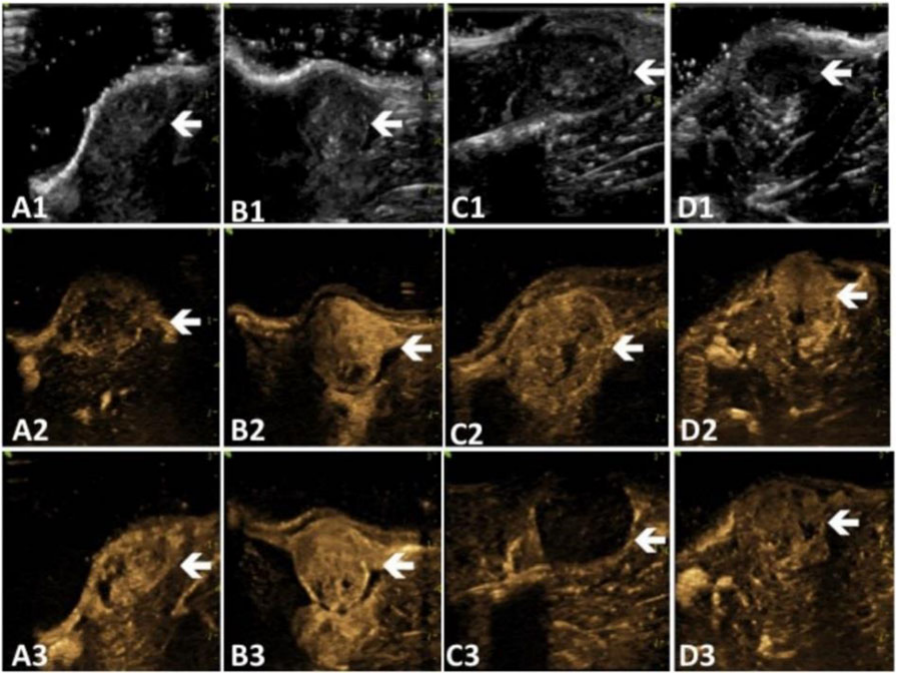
Ultrasound contrast image of VINNO70 before and after the treatment.(A, B, C, D on behalf of MI=0.3,0.7,1.4 group and control group, 1, 2 and 3 respectively represent the two­dimensional ultrasound,ultrasound contrast image before treatment and after treatment) Images show that the group of MI=0.3 combined with microbubbles can enrich the blood perfusion of VX2 tumor in rabbits, MI=1.4 group decrease the blood perfusion,and there was no significant change in the blood perfusion of the MI=0.7group and control group before and after treatment
